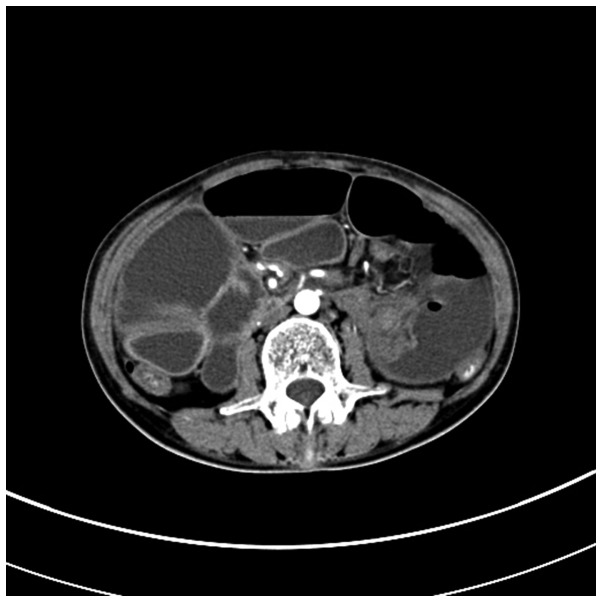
Abdominal computed tomography scan revealing lower intestinal obstruction.
